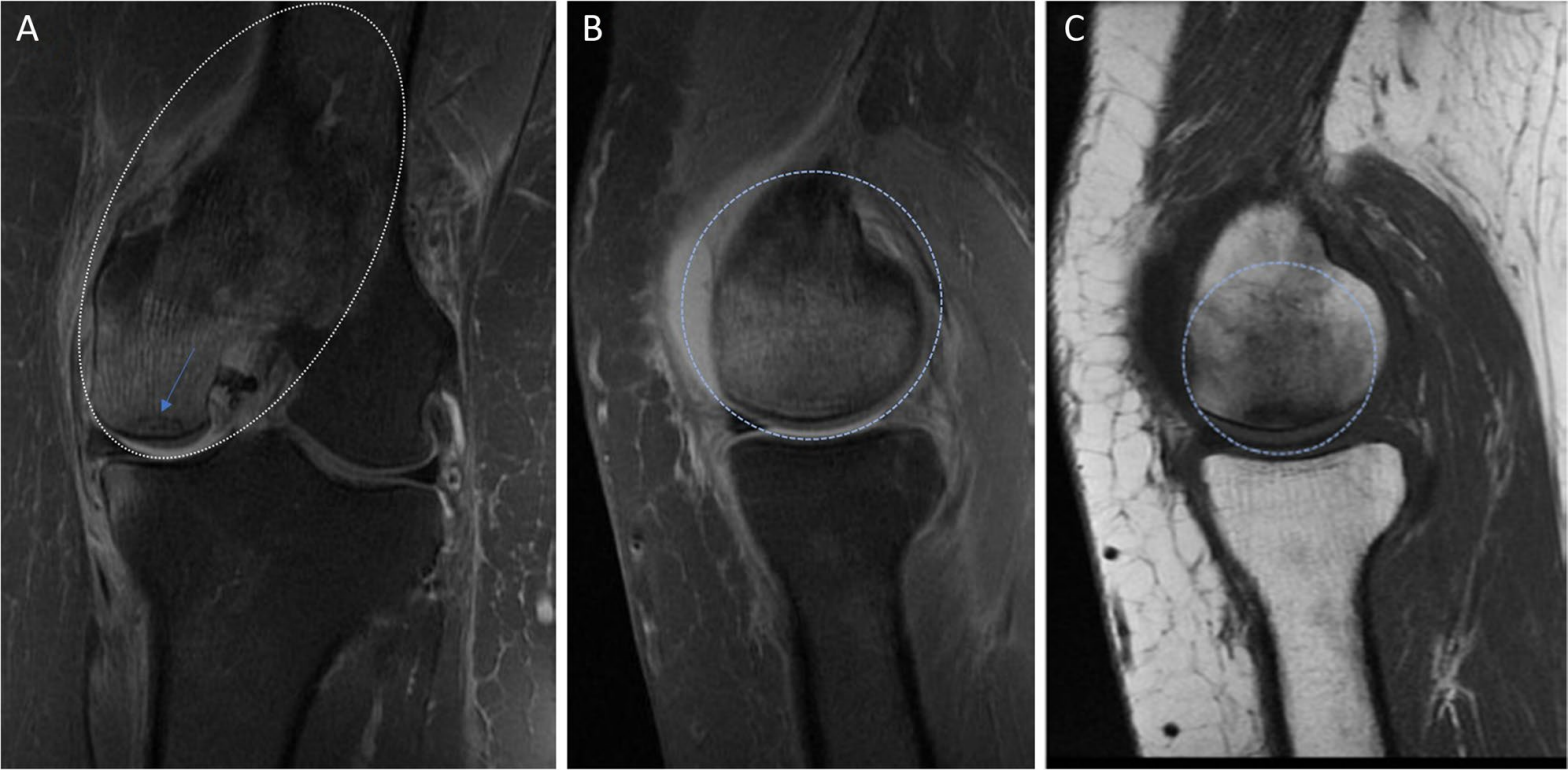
A PD (fat suppressed) coronal image shows extensive oedema in the medial condyle and distal femur metaphysis and diaphysis (dotted ellipse). A discrete subchondral low signal line is also seen (arrow). B PD sagittal image shows oedema in the femur (dotted circle), no certain subchondral line. C T1-weighted sagittal image shows the same oedema and a dark subchondral curved line, which represents a subchondral insufficiency fracture line
